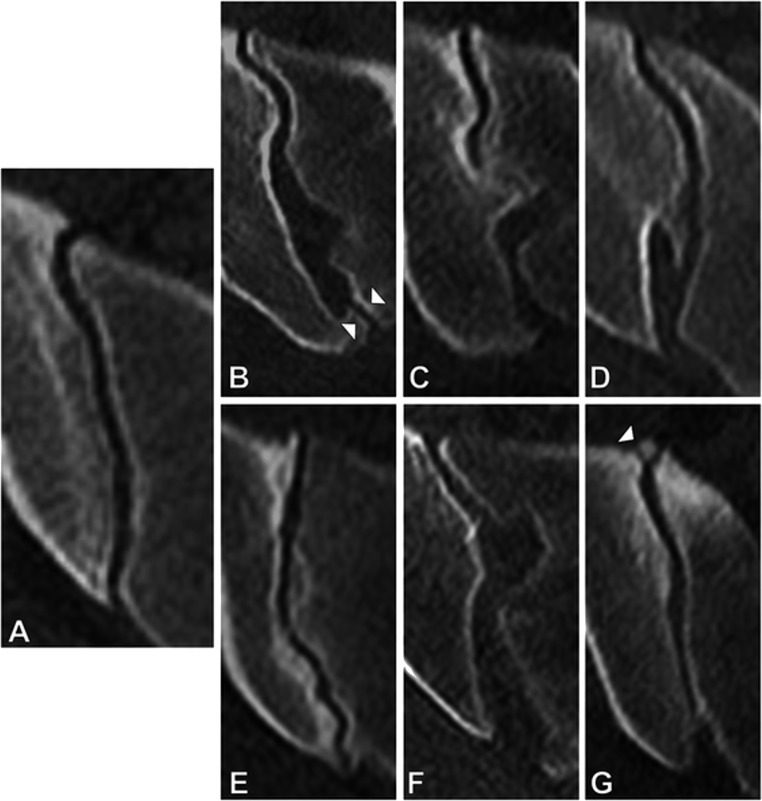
Joint forms. According to the classification by Prassopoulos et al. [21]. Axial reconstructions of computed tomography images. (A) Typical joint. (B) Accessory joint (indicated by white arrowheads). (C) Iliosacral complex. (D) Bipartite ilium. (E) Crescent-shaped ilium. (F) Semi-circular defects. (G) Sacral ossification center (indicated by white arrowhead). Images adapted with the authors’ permission from [22]
